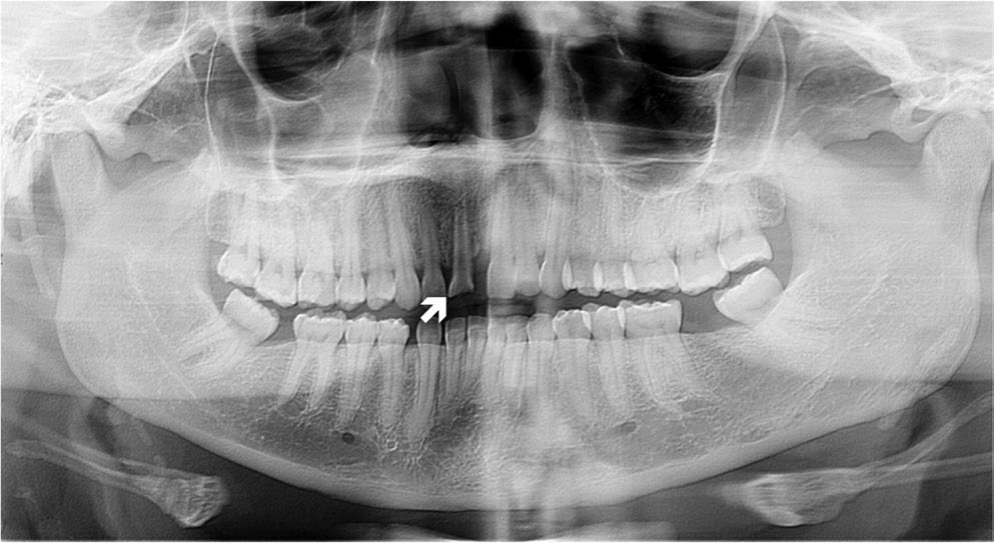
Orthopantomography of a 28-year-old male migrant, history of dental trauma. Fracture of an upper central incisor (arrow). Also note the loss of both lower second molars
